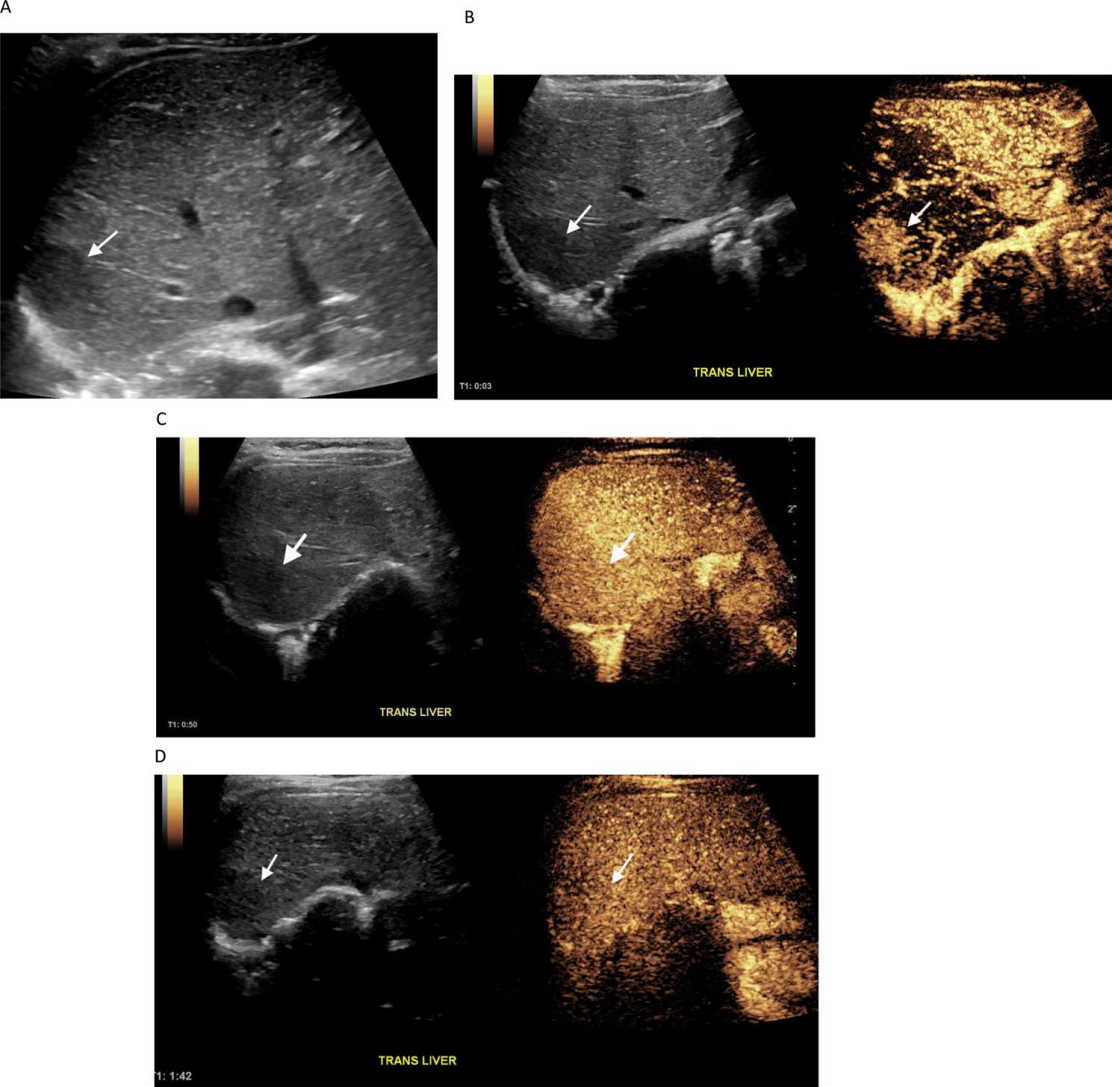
5-month-old female with Trisomy 18 had an (A) Incidentally discovered FLL (arrow) on surveillance ultrasound (B) Arterial-phase CEUS image obtained 3 s after contrast administration shows hyperenhancement of the lesion (arrows). (C) Portal-venous-phase CEUS image obtained at 50 s after injection and (D) delayed-phase CEUS image obtained at 1 min and 42 s both show isoenhancement of the lesion (arrows). The lesion grew on two-month follow-up and was pathologically proved to be hepatoblastoma
